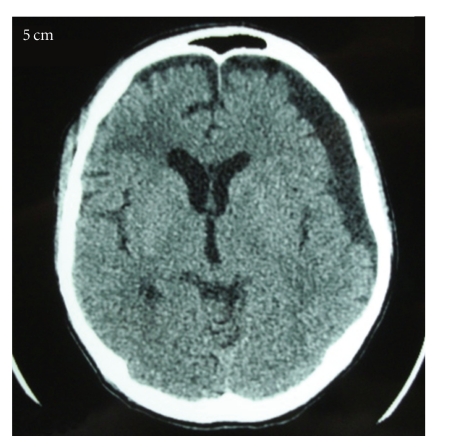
CT scan showing an increasing subdural fluid collection with mild mass effect and some effacement of lateral ventricle.
